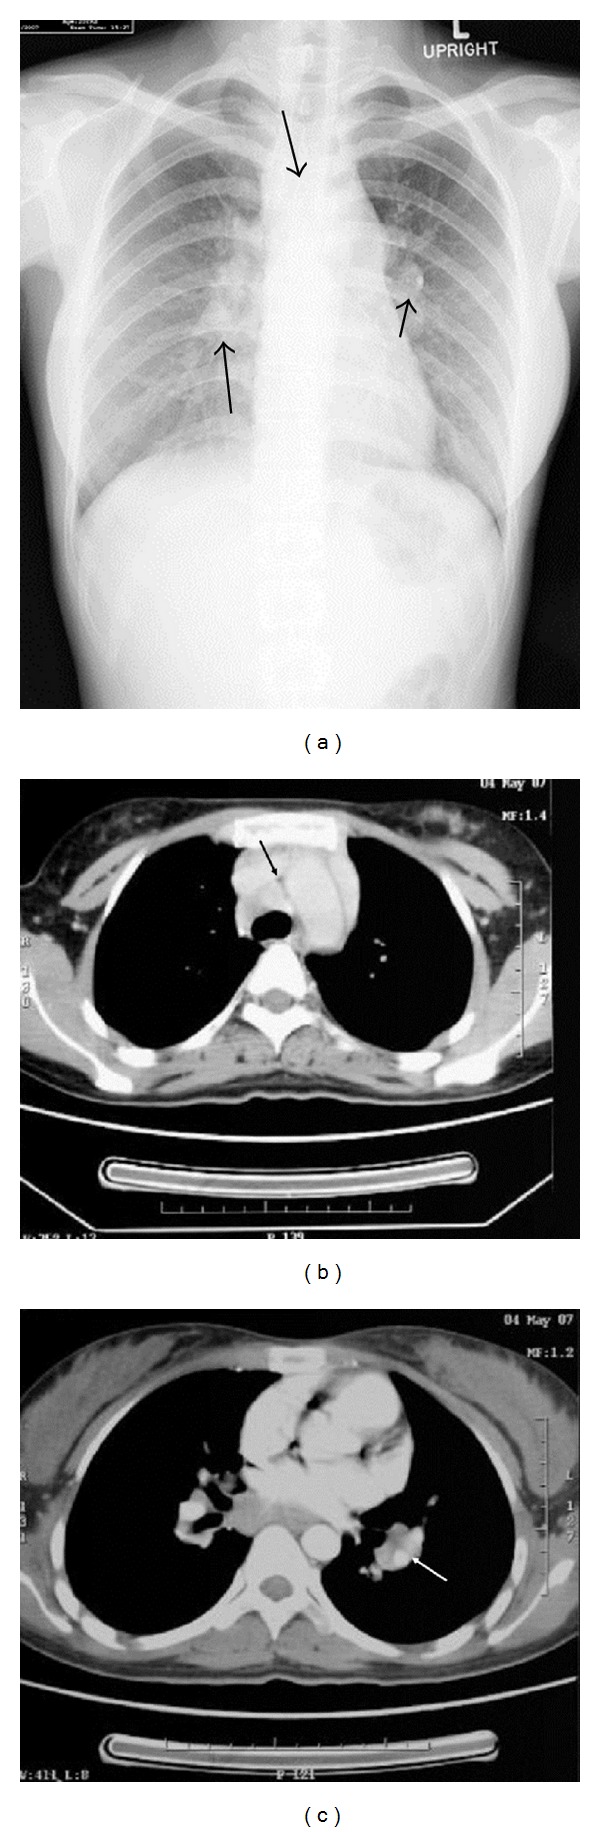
(a) Film chest PA showing widening mediastinum (5.9 cm), bilateral hilar enlargement sizes 2.1 × 6.4 cm and 2.9 × 6.2 cm. Arrows point to mediastinal nodes and both hilar nodes. The lung parenchyma was normal. (b) and (c) CT with contrast showing paratracheal nodes, subcarinal nodes, and bilateral hilar nodes.
